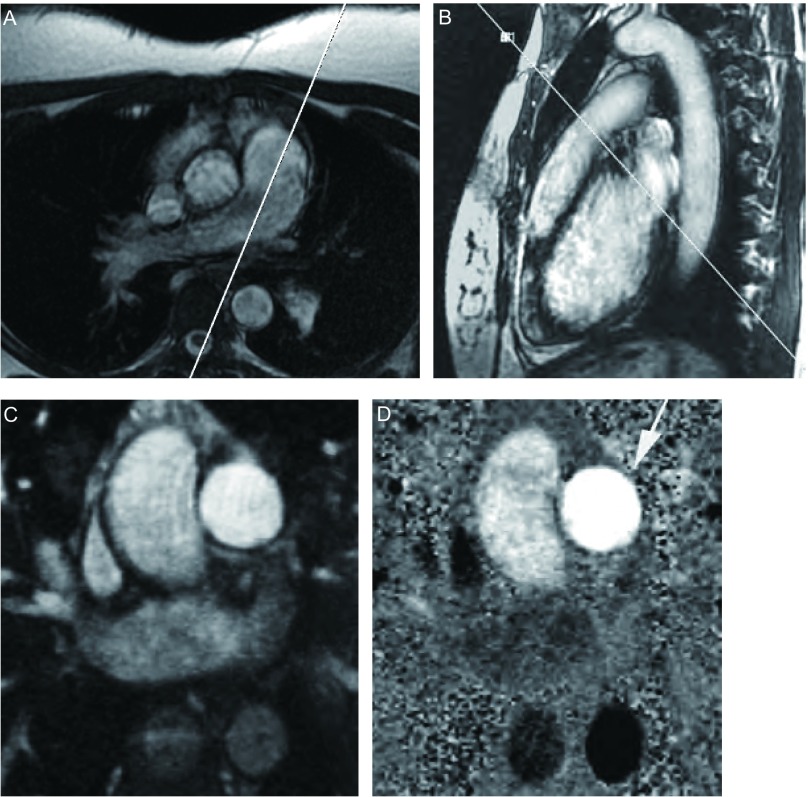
MPA的PC-MRI血流测量。在轴位图像上取平行于MPA走行方向（A）扫描得到MPA长轴图（B），在长轴图肺动脉瓣上方1.5 cm-2 cm处取垂直于MPA长轴方向扫描，得到相应的幅度图（C）和相位图（D）。MPA（箭）血流方向与流速编码方向一致，显示为亮信号。 Flow measurement in MPA by PC-MRI. The longaxis image of MPA (B) was prescribed parallel to the MPA long-axis in the axial image (A). An image plane was positioned perpendicular to the long-axis image of MPA, 1.5 cm-2 cm above the level of the pulmonary valve, resulting in magnitude (C) and phase-map images (D). Flow direction in MPA was the same with the velocity encoding direction, flow in MPA (arrow) was displayed as white.
